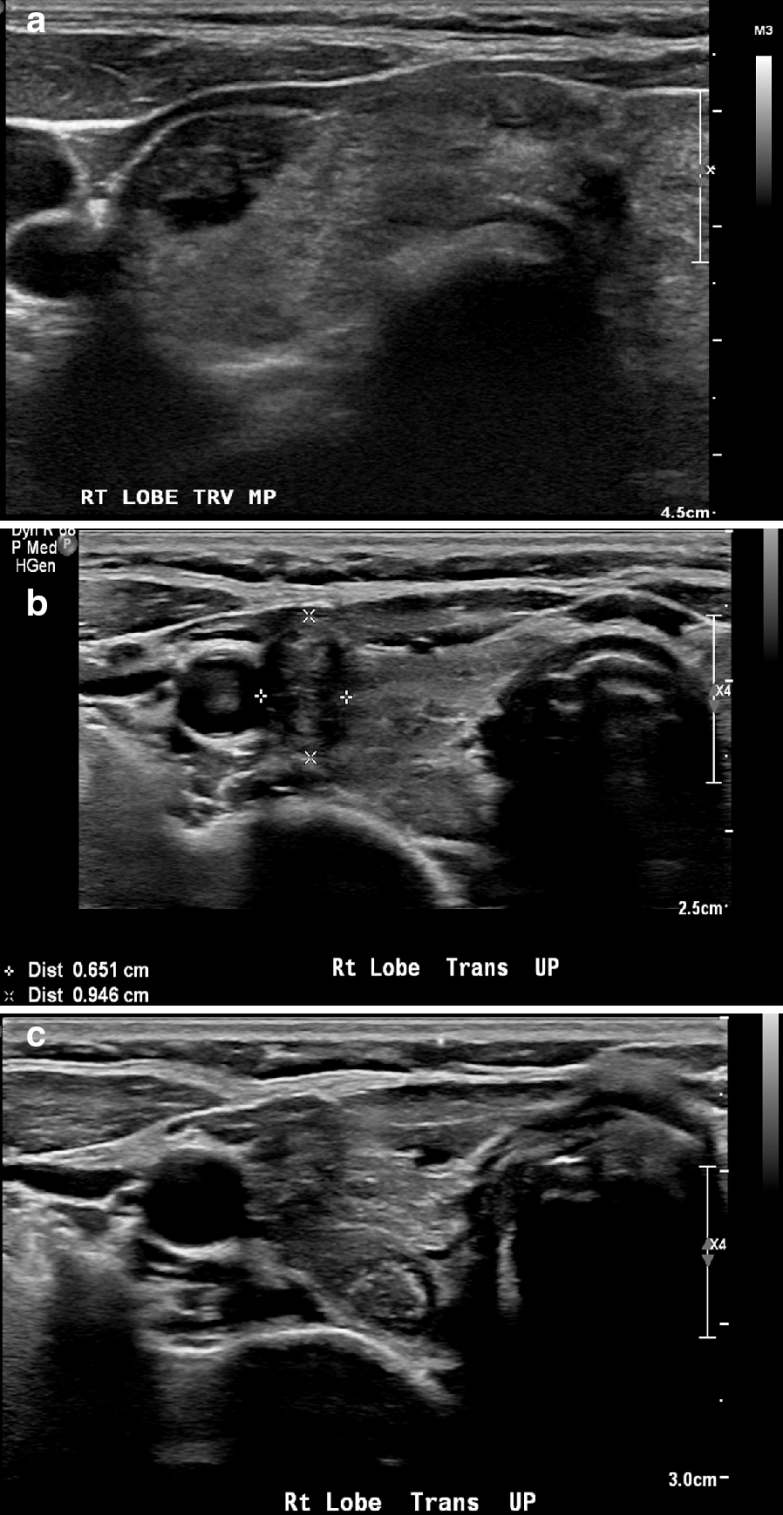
Multiple transverse B-mode ultrasound images obtained by linear probe examination showing: a Well-defined hypoechoic nodule with irregular outline within the right lobe of the thyroid gland. b Well-defined non-homogenous hypoechoic, taller-than-wide; smooth outline nodule is seen within the right thyroid lobe. It is not surrounded by a halo and does not contain internal calcification. c Well-defined isoechoic nodule surrounded by hypoechoic halo with regular outline within the posterior aspect of the right lobe of the thyroid gland
